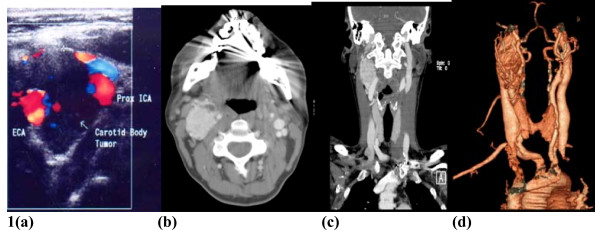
A: Duplex US images of the neck demonstrating soft tissue masses between the left ICA and left ECA. 1B, 1C, 1D: Axial CT post IV contrast and coronal 3D reconstruction showing right sided neck mass extending from the jugular foramen to the level of the mandible.
